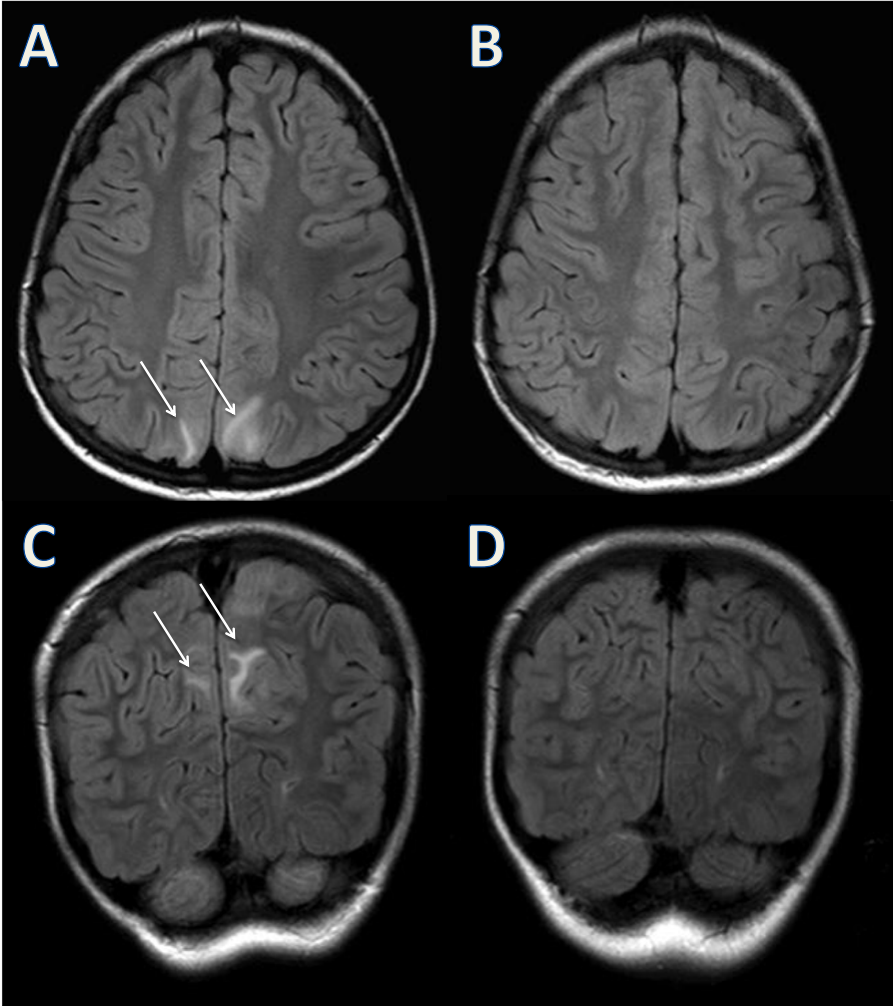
Brain MRI parietal features. MRI fluid attenuated inversion recovery (FLAIR) T2 images on axial (A) and coronal planes (C) showing high signal intensities in parasagittal subcortical parietal regions (indicated by the arrows). Follow up after 6 days showing a complete resolution of the lesions on axial (B) and coronal planes (D).
